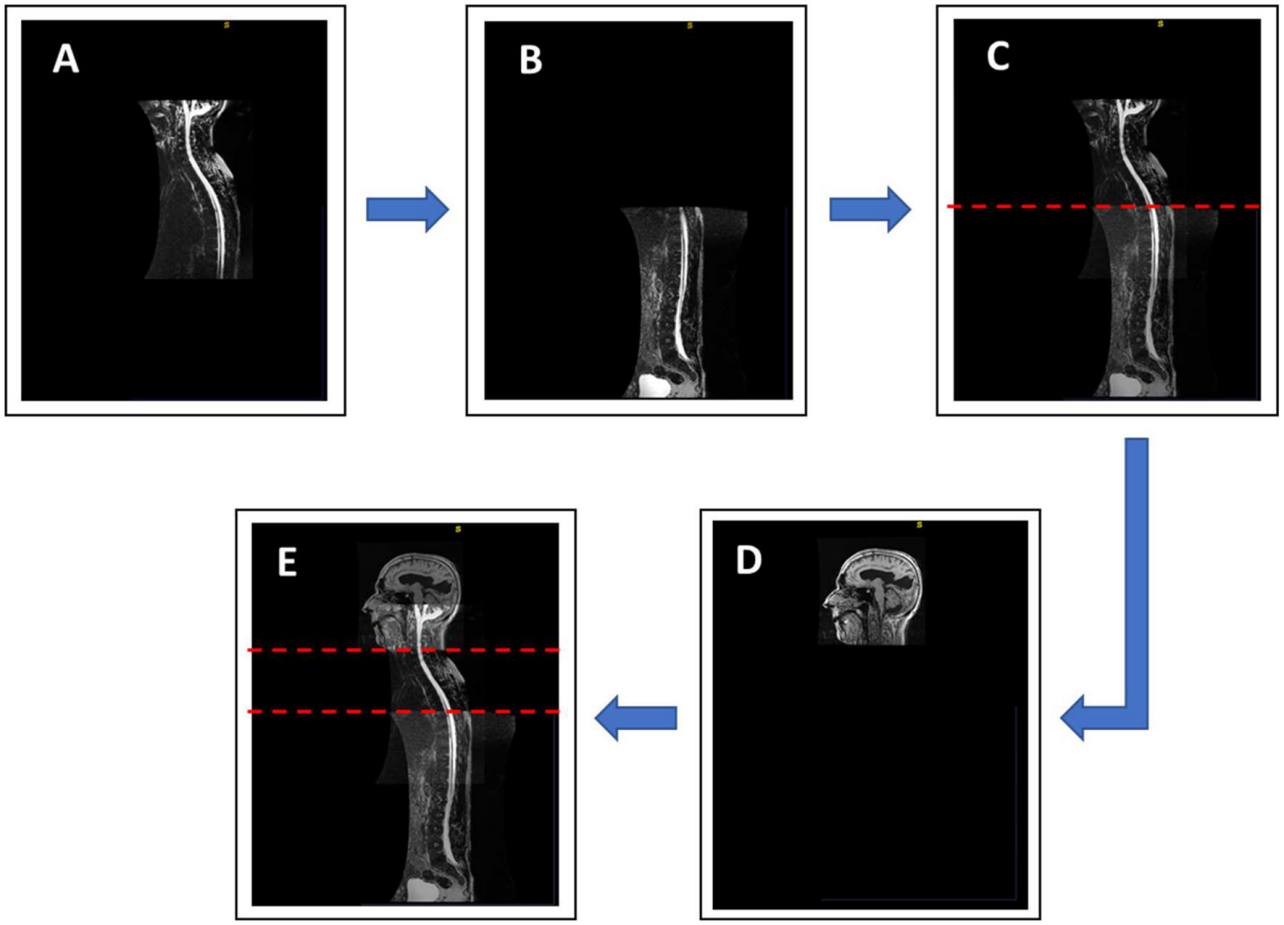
Sagittal image (E) showing complete coverage of the neural axis, obtained by MRI superposition and fusion of three different regions: cranial T1 images (D), cervicothoracic (A) and thoracolumbar (B) T2 images of spinal segments. (C) Image of the spine obtained by fusion of two recorded segments of the spine. The red dashed lines show overlapping of individual segments.
